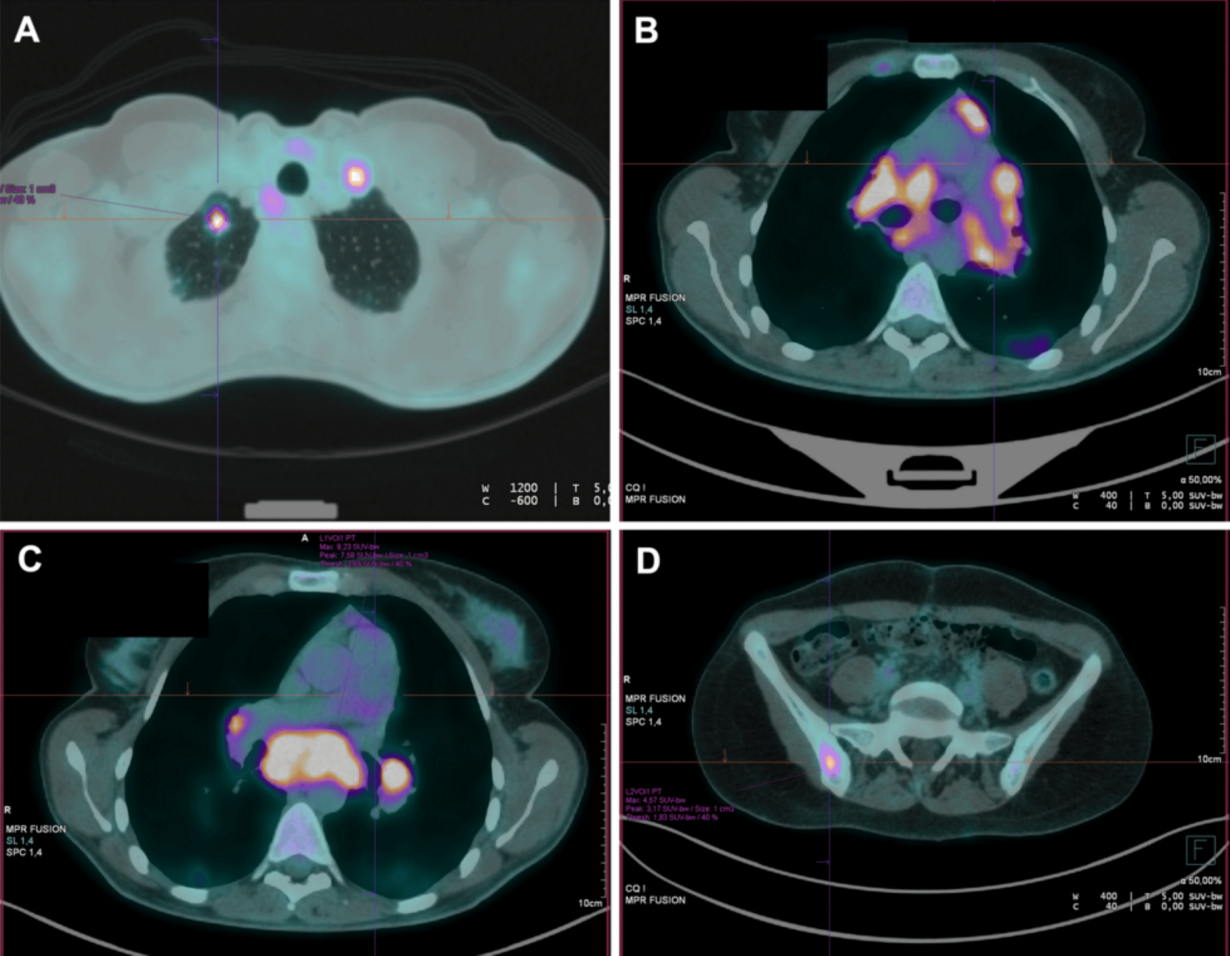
Baseline ¹⁸F-FDG PET/CT images showing (A) hypermetabolic pulmonary nodules in the right upper lobe (SUVmax = 9.78), (B, C) mediastinal and hilar lymphadenopathy (SUVmax = 9.23), and (D) intense focal uptake in the right iliac bone (SUVmax = 11.22). ¹⁸F-FDG PET/CT: fluorine-18 fluorodeoxyglucose positron emission tomography/computed tomography
